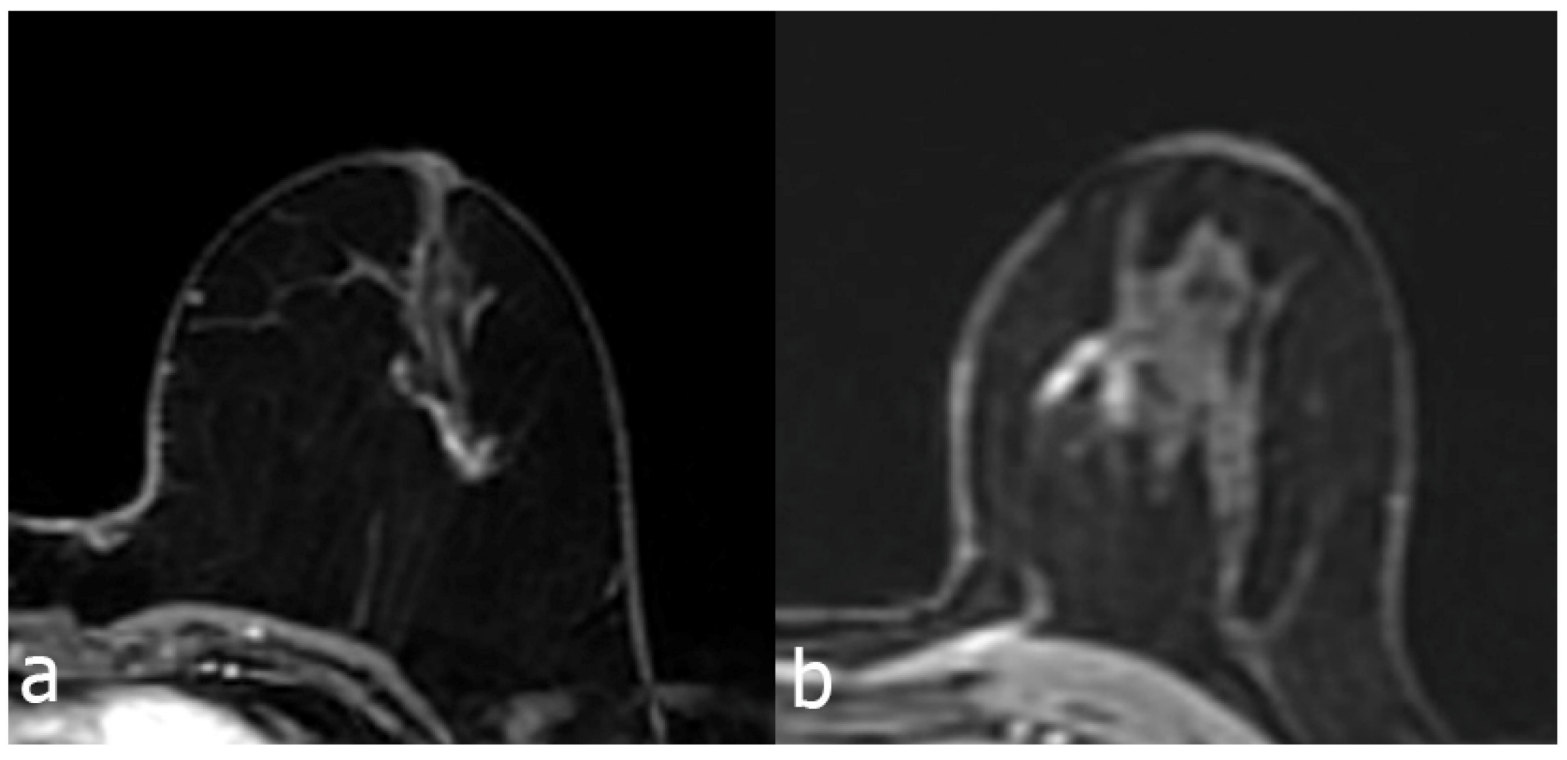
Contrast enhanced breast MRI, linear heterogeneous branching type enhancing NME lesion (a) 56 years old, atypical ductal hyperplasia due to biopsy. Similarly, in a 44-year-old patient with NME lesion showing linear heterogeneous branching contrast enhancement (b), the biopsy result was DCIS.
